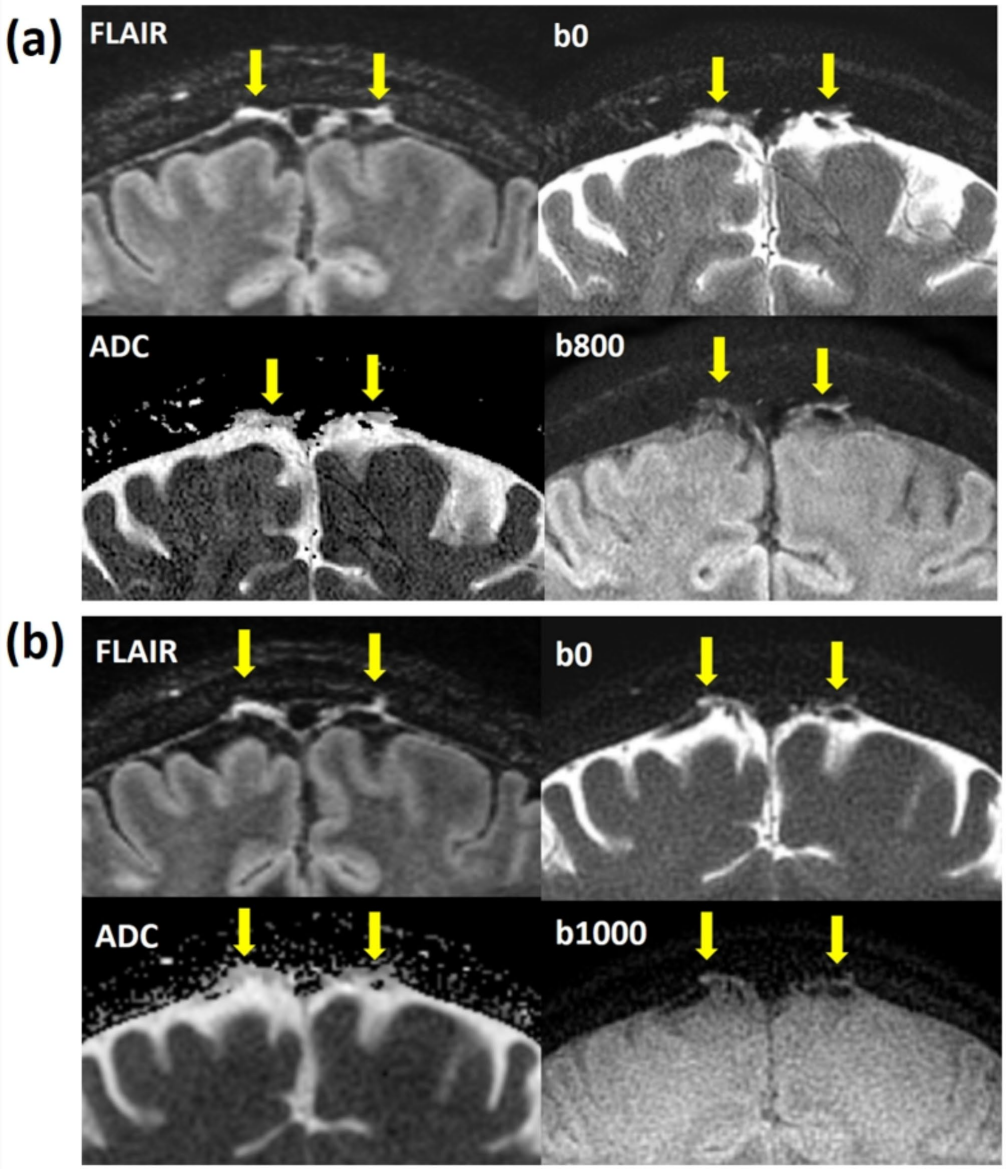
(a) The coronal view displayed the PSD on T2 FLAIR image (up left), MUSE DWI b0 (up right), MUSE DWI b800 (down right), and MUSE ADC map (down left) as indicated by arrows. (b) The coronal view displayed the PSD on T2 FLAIR image (up left), PROPELLER DWI b0 (up right), PROPELLER DWI b1000 (down right), and PROPELLER ADC map (down left) as indicated by arrows. The ADC of the PSD appeared darker than that of the CSF.
